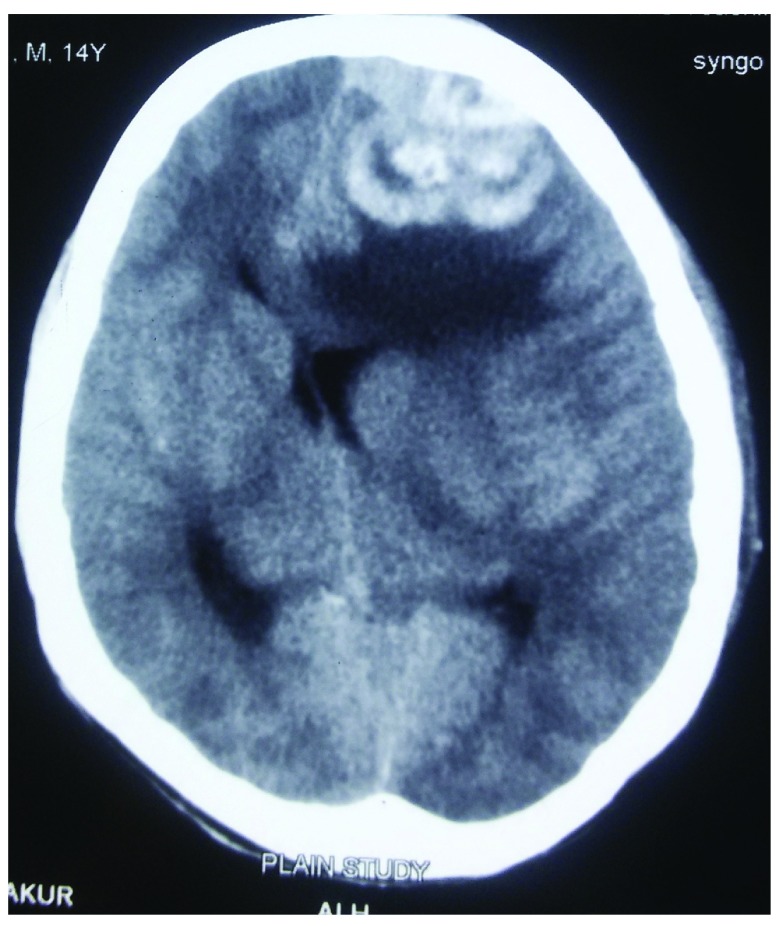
CT image showing the herniation syndrome with gross mass effect.
